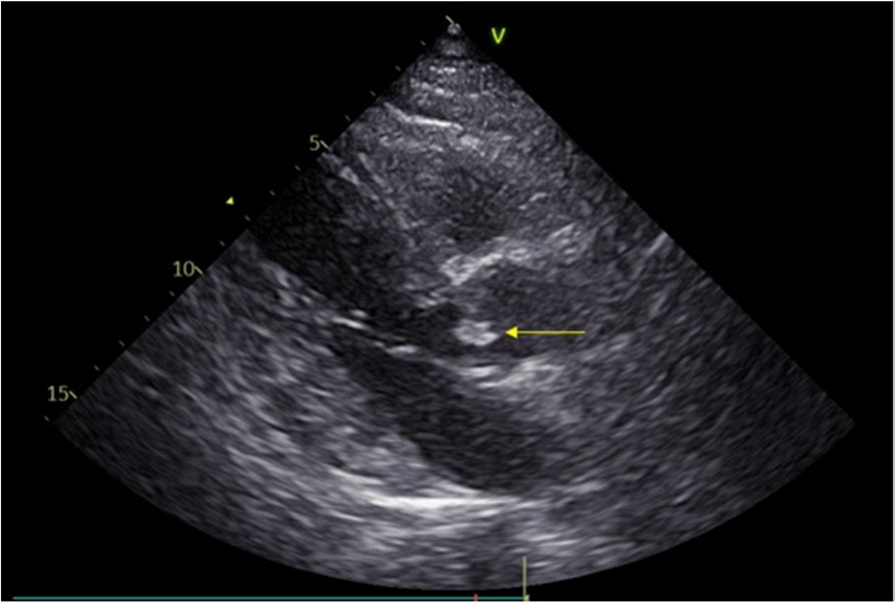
Transthoracic echocardiogram shows a mobile echodensity on the anterior aortic leaflet measuring 20 x 14 mm consistent with endocarditis (yellow arrow)
